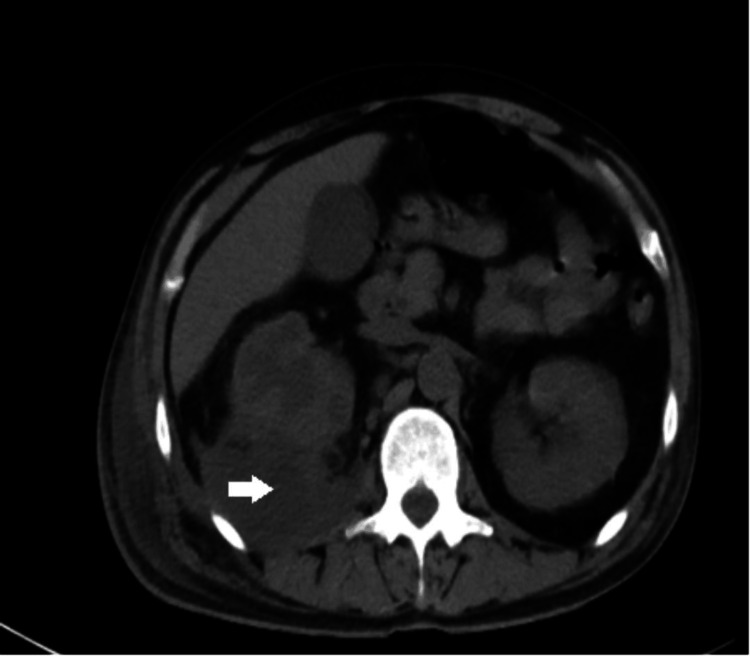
CT abdomen (axial view) - right perinephric abscess (white arrow)
